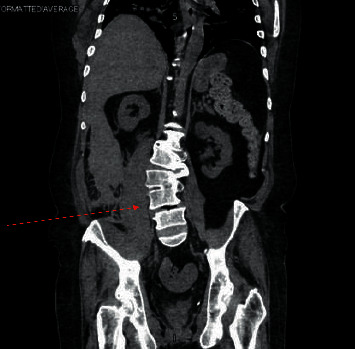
CT abdomen pelvis coronal view: large right-sided retroperitoneal hematoma extending along the psoas musculature and the lateral abdominal wall.
